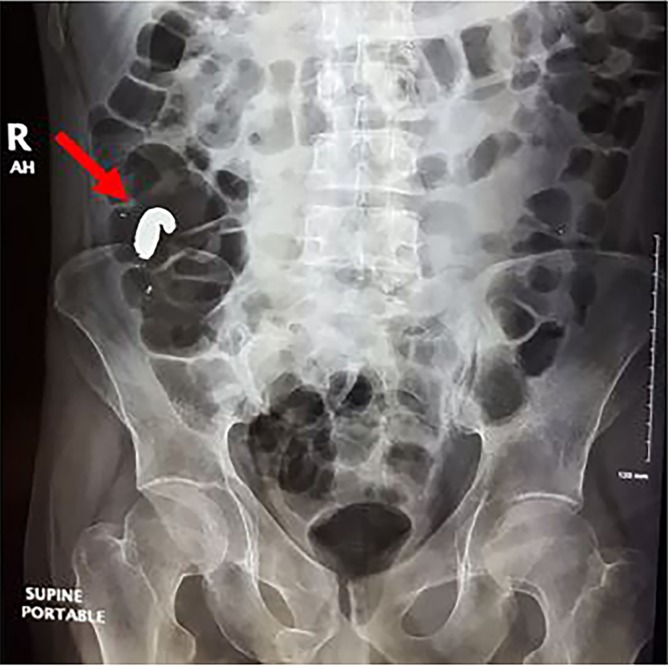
Abdominal radiograph demonstrating high-density material in the right hemi-colonic region (red arrow), which was confirmed to be in the appendix on computed tomography.
